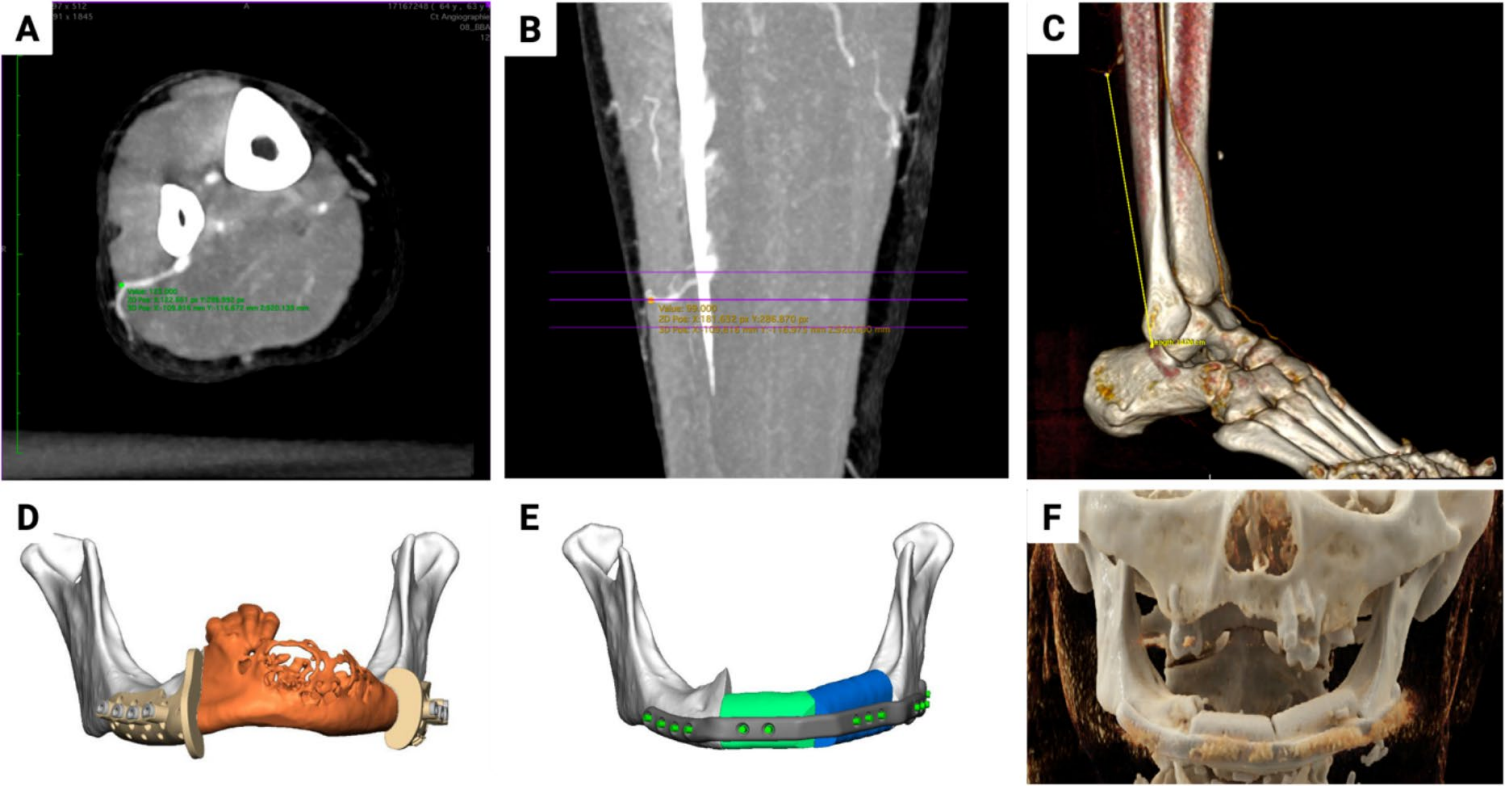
Example of mandibular reconstruction with FFF consisting of two bone segments. (A) Visualization of the SCP on CTA in the axial cross-sectional images. (B) Visualization of the SCP on CTA in the coronal cross-sectional images using MIP. (C) Visualization of the SCP in cinematic rendering. (D) Visualization of the VSP with the orange marked resection area with osteodestructive lesion and the virtually modeled surgical guides. (E) VSP showing the planned reconstruction with two bone segments and modeling of the PSI. (F) Postoperative control CT with cinematic rendering showing the reconstruction result
